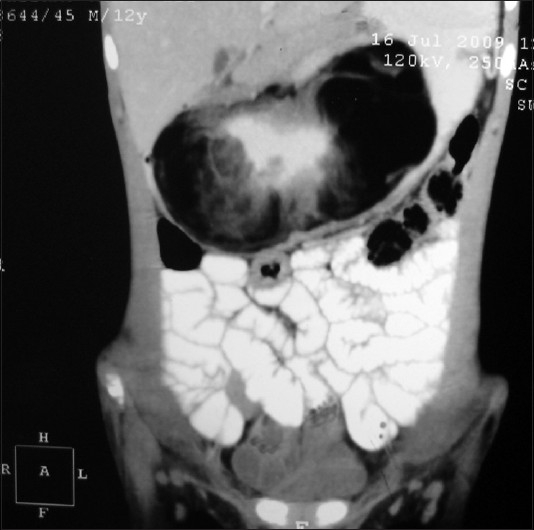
CT abdomen showing a large epigastric mass
